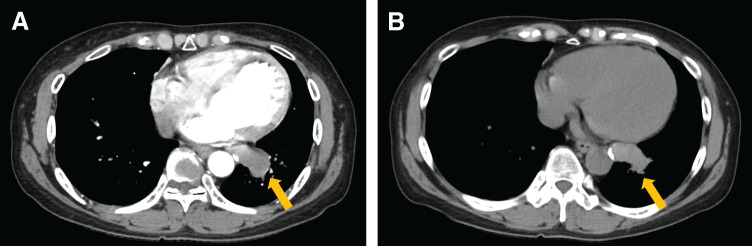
Preoperative chest CT. (A) Contrast-enhanced chest CT showing a 33 mm, irregularly shaped saccular aneurysm arising from an aberrant artery supplying the left basal segment (arrow). (B) Non-contrast chest CT showing a hyperdense lumen within the aneurysm (arrow), suggestive of acute thrombus formation.
